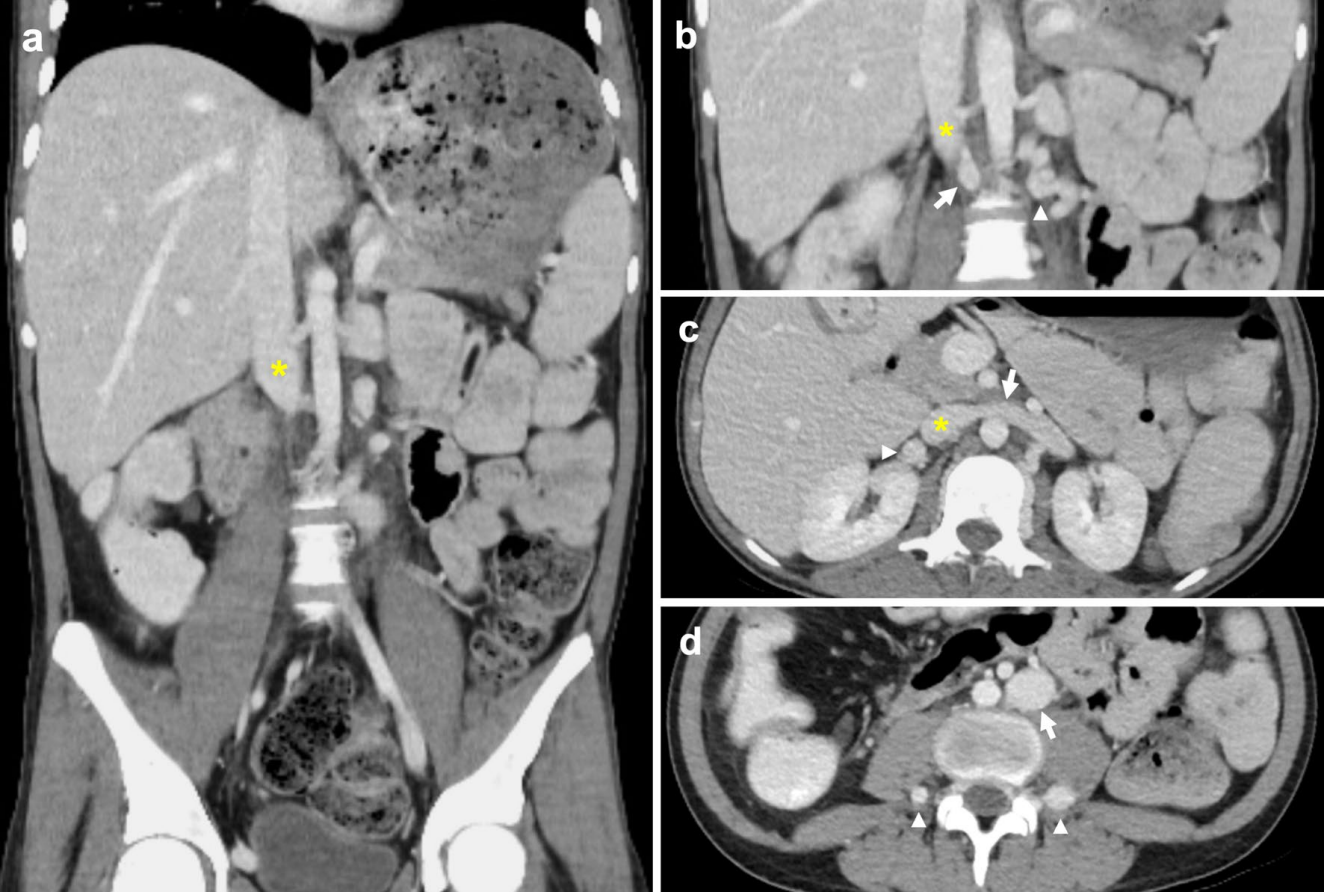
Absent infrarenal IVC with azygos continuation. Paired common iliac veins continue cranially as bilateral ascending lumbar veins. a Contrast-enhanced coronal CT demonstrates the caudal end of the IVC (asterisk). b Coronal CT shows the right ascending lumbar vein (arrow) approaching and subsequently draining into the IVC (asterisk). Tortuous paravertebral collateral veins (arrowhead) are present adjacent to the aorta. c Axial CT image with left renal vein (arrow) crossing anterior to the aorta to drain into the renal IVC segment (asterisk). Right renal vein (arrowhead) also approaches renal IVC segment. d Axial CT shows prominent bilateral ascending lumbar veins (arrowheads) and a distended paravertebral collateral (arrow) positioned left of the aorta
